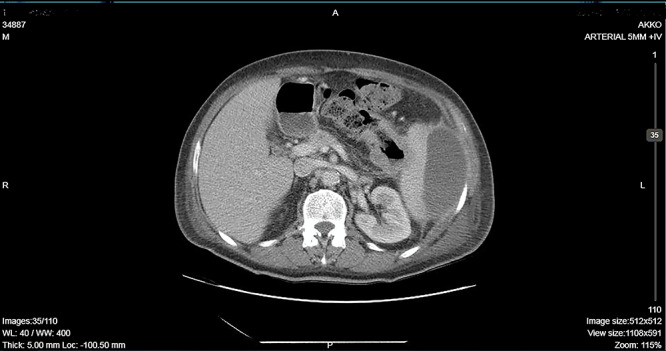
CT showing descending colon wall thickening in contact with the spleen and the tail of the pancreas and a subcapsular splenic abscess as well as splenic vein thrombosis.
